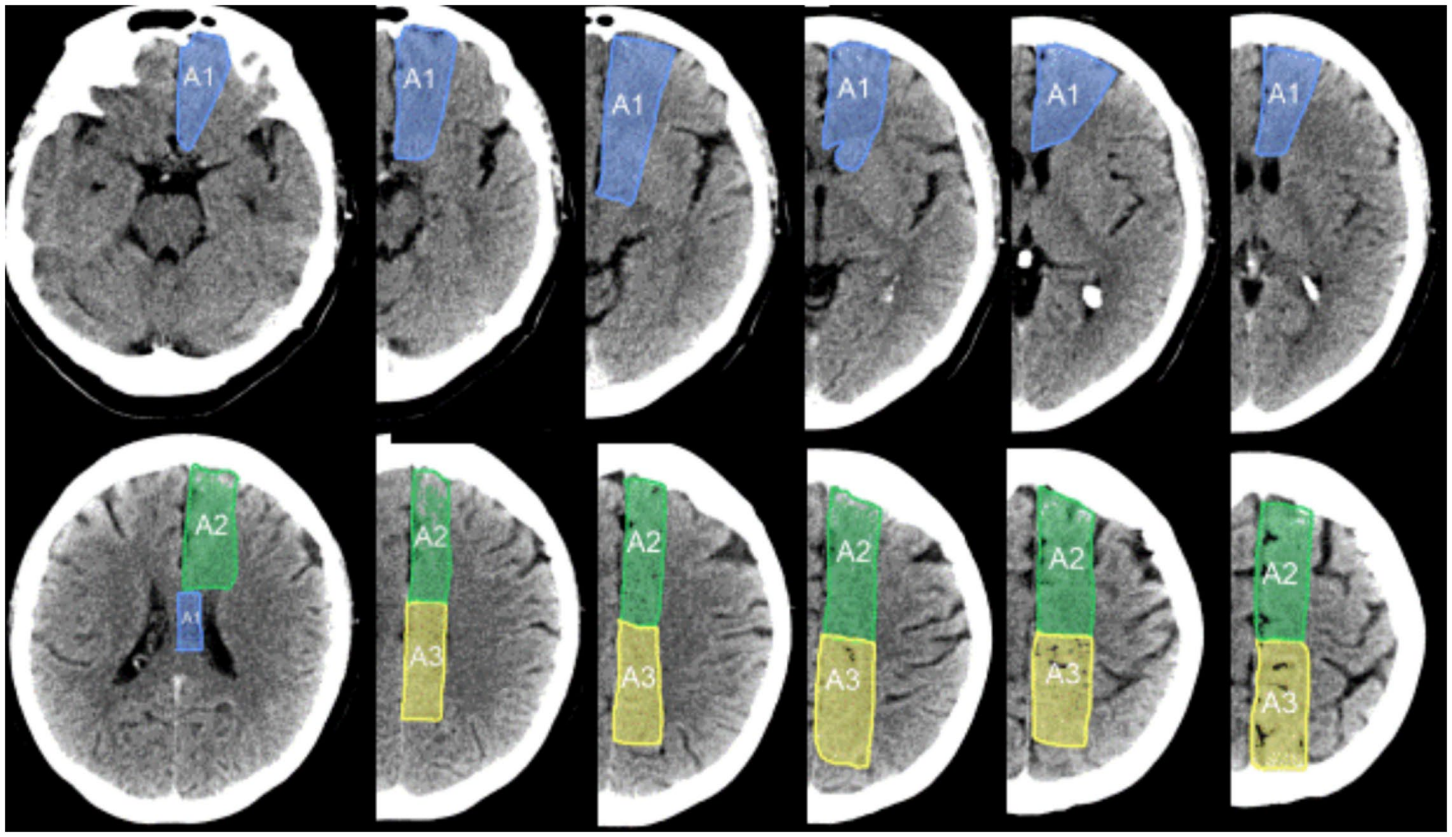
AC-ASPECTS subregions within the anterior cerebral artery territory. The A1 region is the inferior portion of the ACA territory, extending from the lowest slice through the ACA territory up to the highest slice that includes the lateral ventricles. On the highest cut, the A1 region lies only between the ventricles. The A2 region is the anterosuperior portion of the ACA territory. Along the vertical axis, it starts at the highest cut through the lateral ventricles and continues to the highest cut through the top of the cerebrum. Along the anteroposterior axis, the A2 region is the anterior half of the ACA territory on each slice. The A3 region is the posterosuperior portion of the ACA territory. Like the A2, along the vertical axis, it starts at the highest cut through the lateral ventricles and continues to the highest cut through the top of the cerebrum. Along the anteroposterior axis, the A3 region is the posterior half of the ACA territory on each slice.
